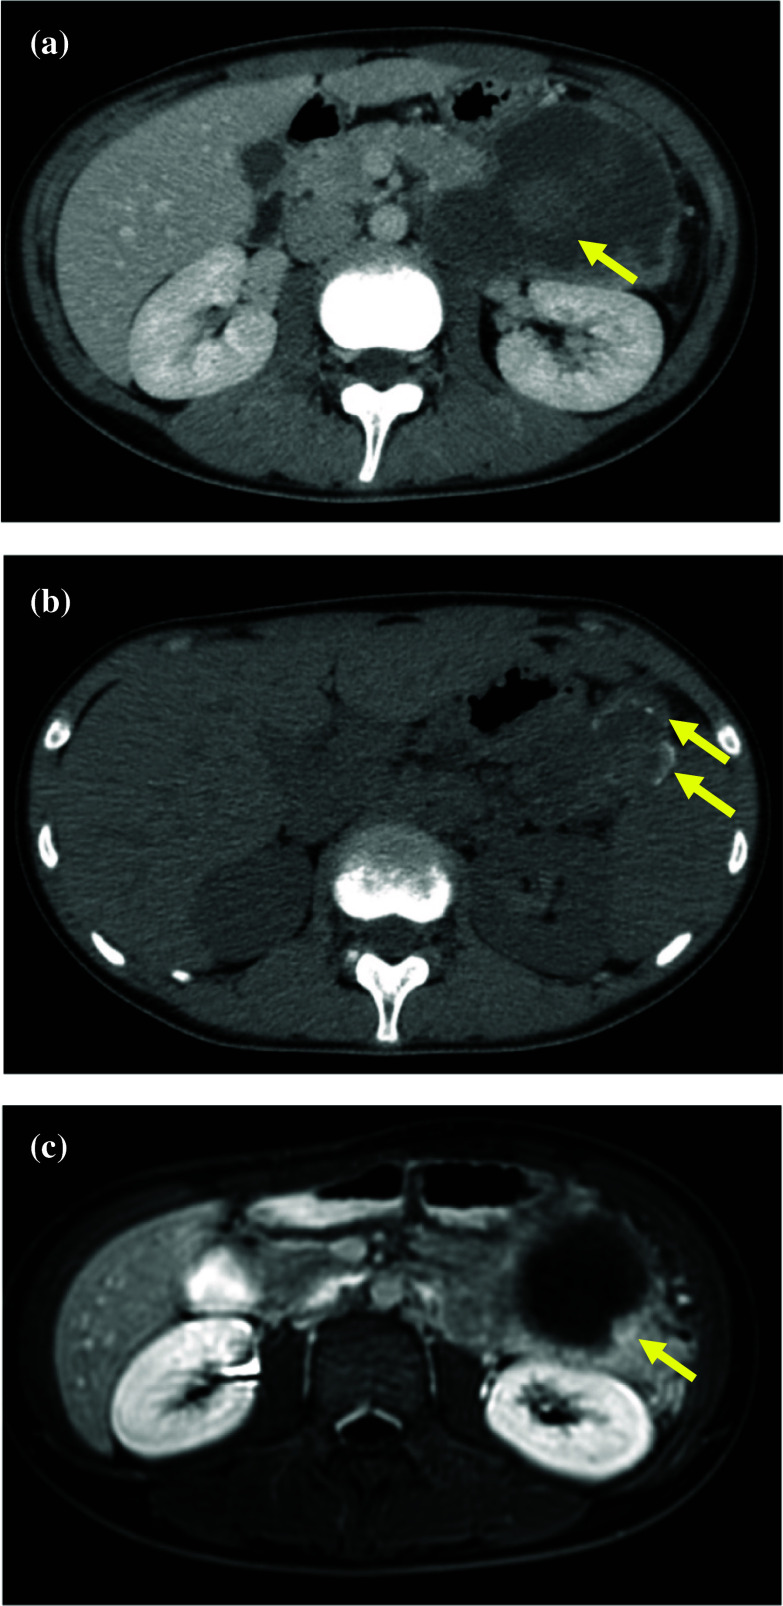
a Enhanced CT. An 8.3 × 5.3 × 7.1 cm cystic pancreatic tail tumor with hemorrhage (yellow arrow). b Plane CT. The pancreatic tail tumor partially contained calcifications (yellow arrow). c MRI T2-weighted image. A nodular enhancement inside the mass was suggested a high cellular density lesion (yellow arrow)
